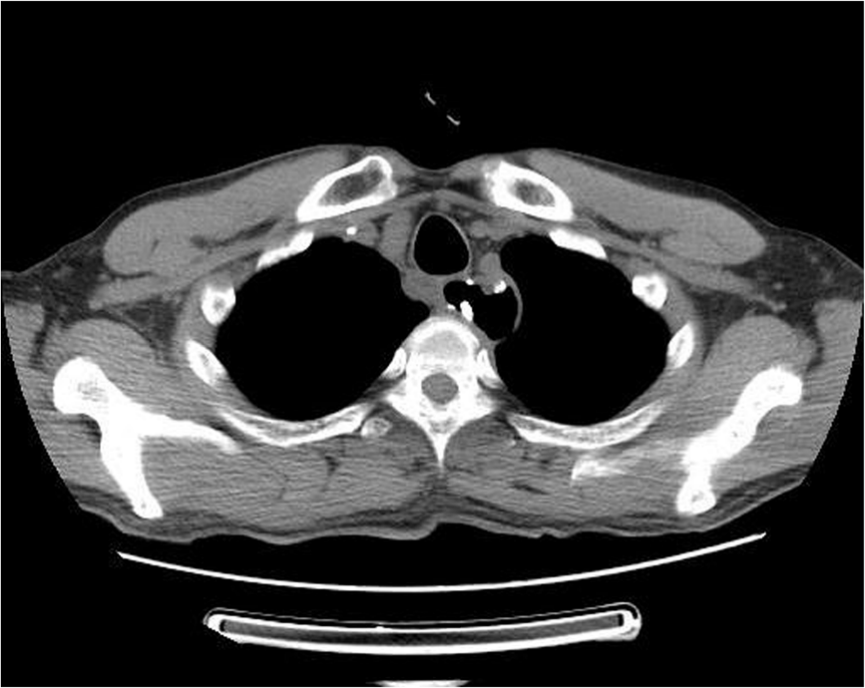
Oral contrast computed tomography was repeated after 5 weeks. No extraluminal contrast and air were found. Closure of the anastomotic leak was confirmed by the following oral feed.
